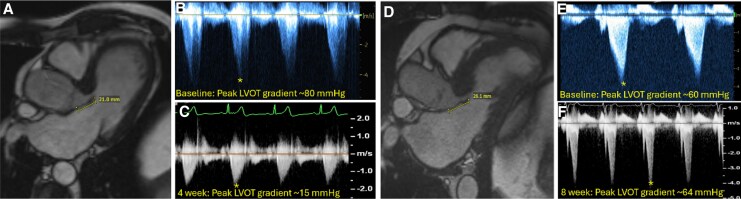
Case examples of a rapid responder (*A–C*) and a late responder (*D–F*) to mavacamten. (*A*) AMVL length (21 mm) measured by cMRI in mid diastole; (*B*) Peak LVOT gradient from baseline Echocardiogram; (*C*) Peak LVOT gradient after 4-weeks of Mavacamten 5 mg daily; (*D*) AMVL length (26.1 mm) measured by cMRI in mid diastole; (*E*) Peak LVOT gradient from baseline Echocardiogram; (*F*) Peak LVOT gradient after 8-weeks of Mavacamten 5 mg daily. *Peak velocities marked with asterisk.
